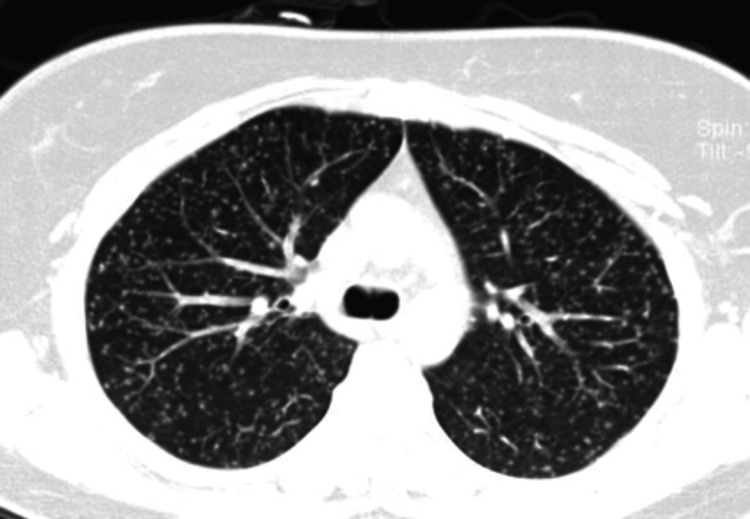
CT scan showing the diffuse micronodular hyperdensities also known as miliary findings of tuberculosis.
